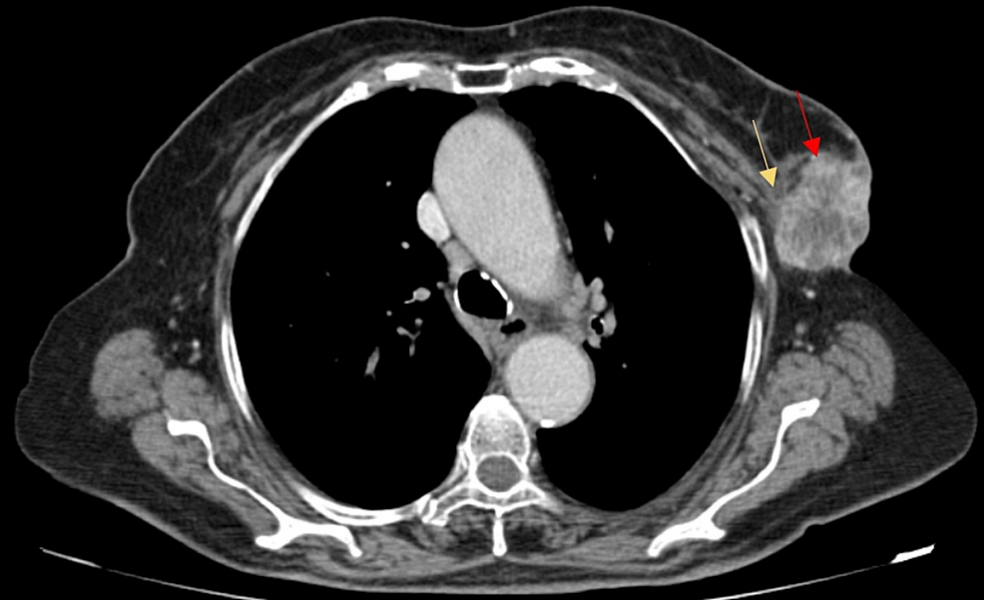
Axial enhanced CT acquisition of the thorax showing a lesion (red arrow) extending to the overlying skin and contacting deeply with the pectoral muscles (yellow arrow), yet without invading the endothoracic fascia. CT, computed tomography
